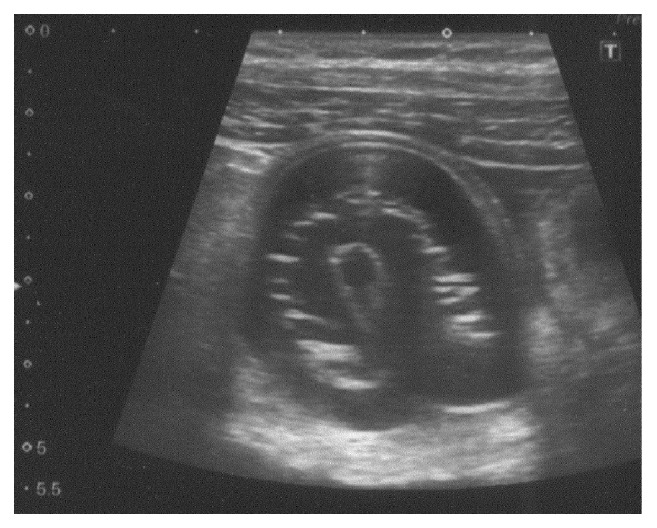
Abdominal ultrasonography revealed an apple-shaped foreign body.
